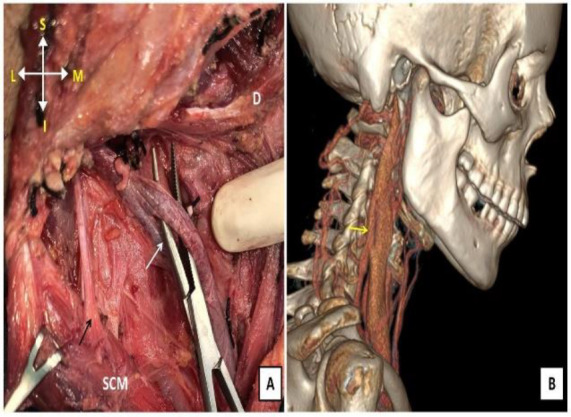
A. Empty fenestration of the right internal jugular vein (white arrow). The spinal accessory nerve is passing lateral to the IJV above the level of the fenestration (black arrow). The sternocleido- mastoid (SCM) and digastric (D) muscles are also seen in the surgical field. B. Reconstructed virtual CT scan of the patient showing the empty fenestration of the right internal jugular vein (yellow arrow).
